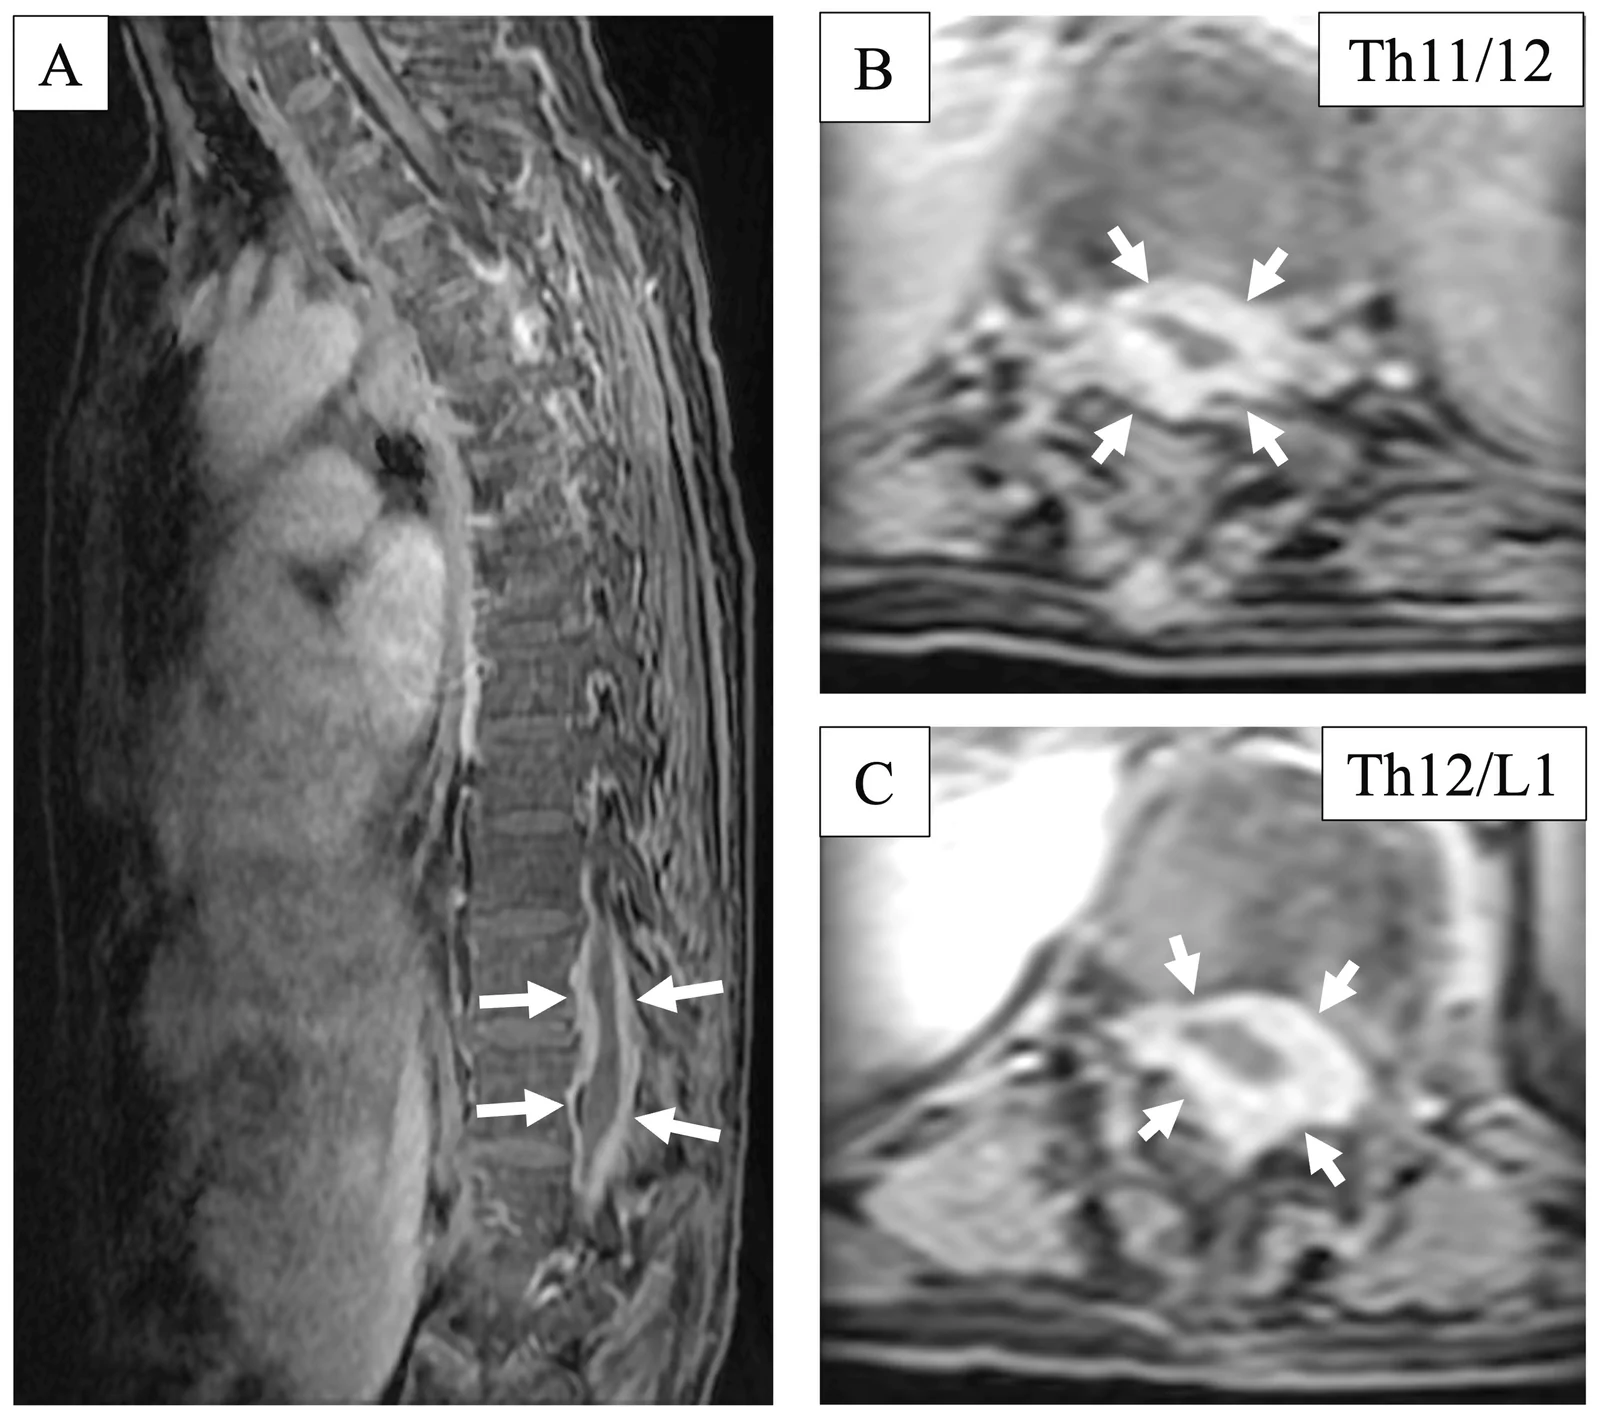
Gadolinium-enhanced MRI on admission to our hospital. (A) Contrast-enhanced sagittal T1-weighted image shows a flattened, contrast-enhancing mass in the spinal epidural space extending from the Th11 to L1 levels (white arrow). (B) Axial image shows an epidural mass compressing the spinal cord at the Th11/12 level (white arrow). (C) Similarly, spinal cord compression was observed at the Th12/L1 level (white arrow).
